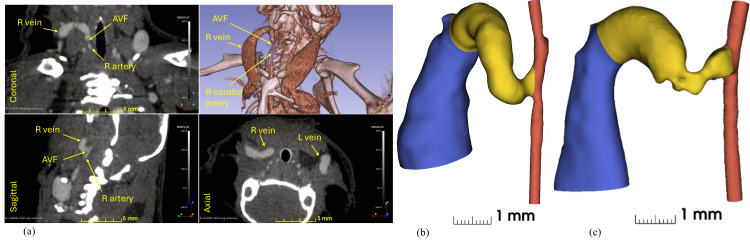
Contrast-enhanced CT images and segmented vascular structure. (a) CT images in three orientations, coronal, sagittal, and axial, near the AVF. A volume rendering of the CT images is shown in the upper right to visualize vasculature and bone structure. The AVF and surrounding veins and arteries are annotated in yellow. (b) and (c) Segmented vasculature near the AVF at day 7 and day 21 post-surgery, respectively. Color coding represents distinct vessel segments: red indicates the carotid artery (with the proximal segment at bottom and distal segment at top), yellow highlights the AVF region (4 mm of length), and blue shows the main venous drainage with its characteristic ballooning of the vein starting 4 mm from the anastomosis.
